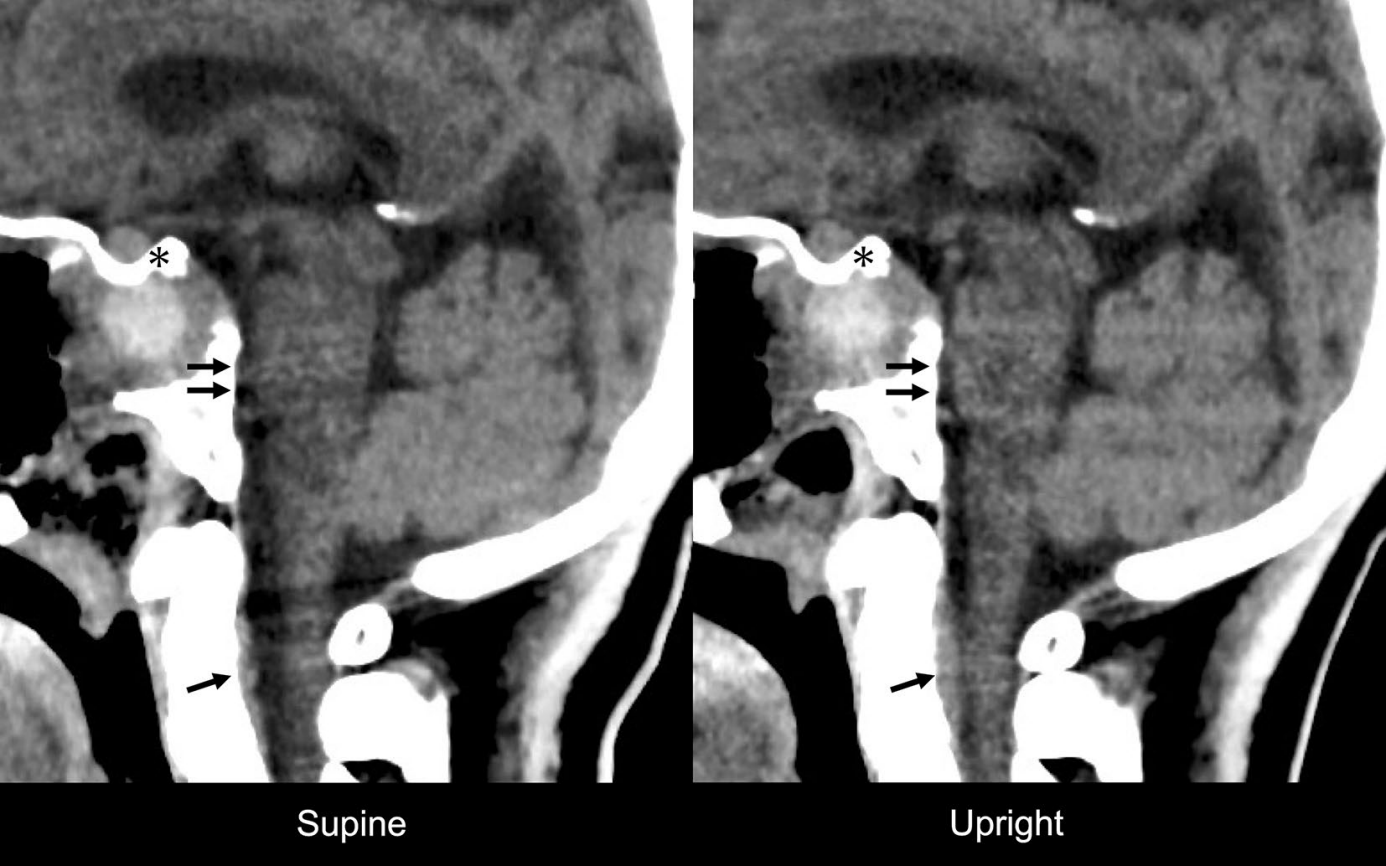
Computed tomography (CT) scan images with 0.5 mm slices of a 35-year-old woman who underwent cerebrospinal fluid leak closure using the endoscopic endonasal approach. Compared with supine imaging, upright CT scan revealed PBS to the ventrocaudal direction, distension of the anterior internal vertebral venous plexus (arrow), effacement of the prepontine cistern (double arrow), and enlargement of the pituitary gland (asterisk). See also Video 1.
